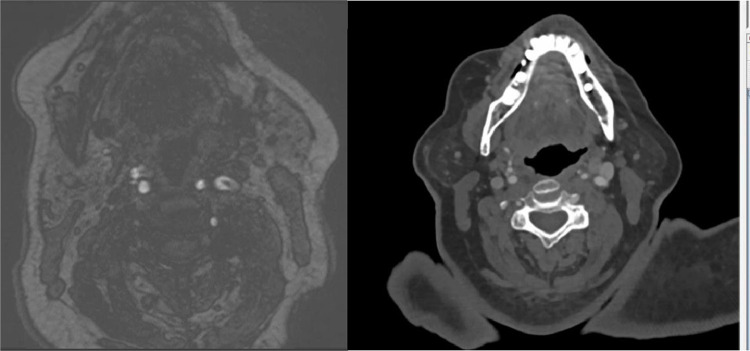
MRA neck (left) demonstrating higher plaque than seen in (1). There is no intraluminal plaque at the same level on CTA performed later, indicating this as the possible source of the dislodged plaque.
